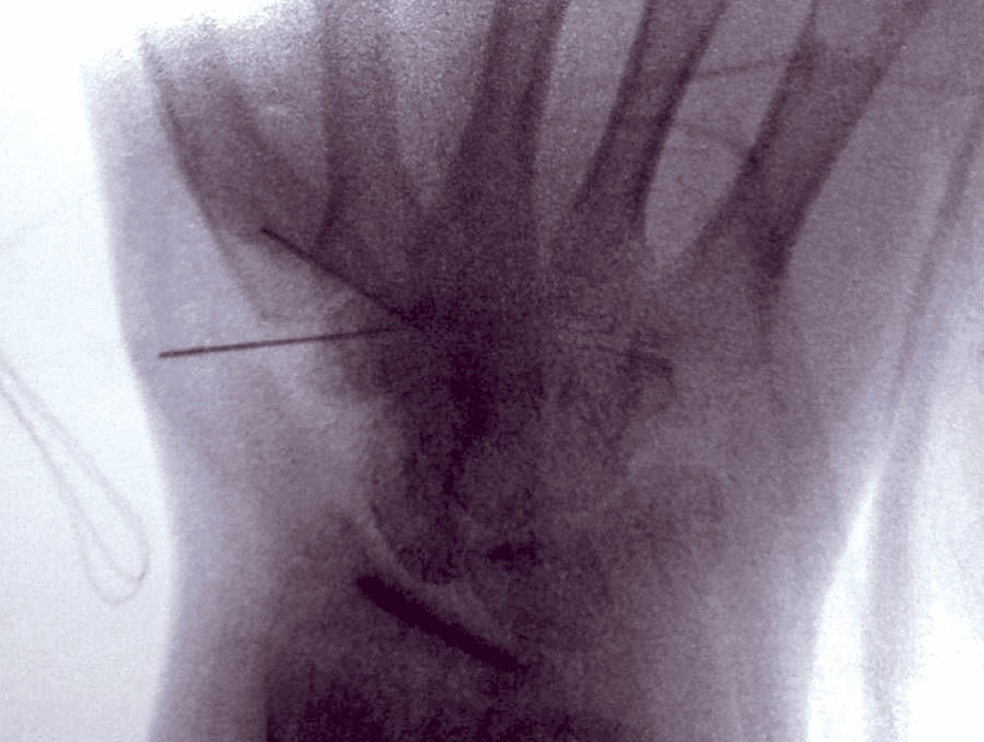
Intra-operative C-arm radiograph The nidus was tagged with two needles and marked with the aid of C-arm fluoroscopy.
